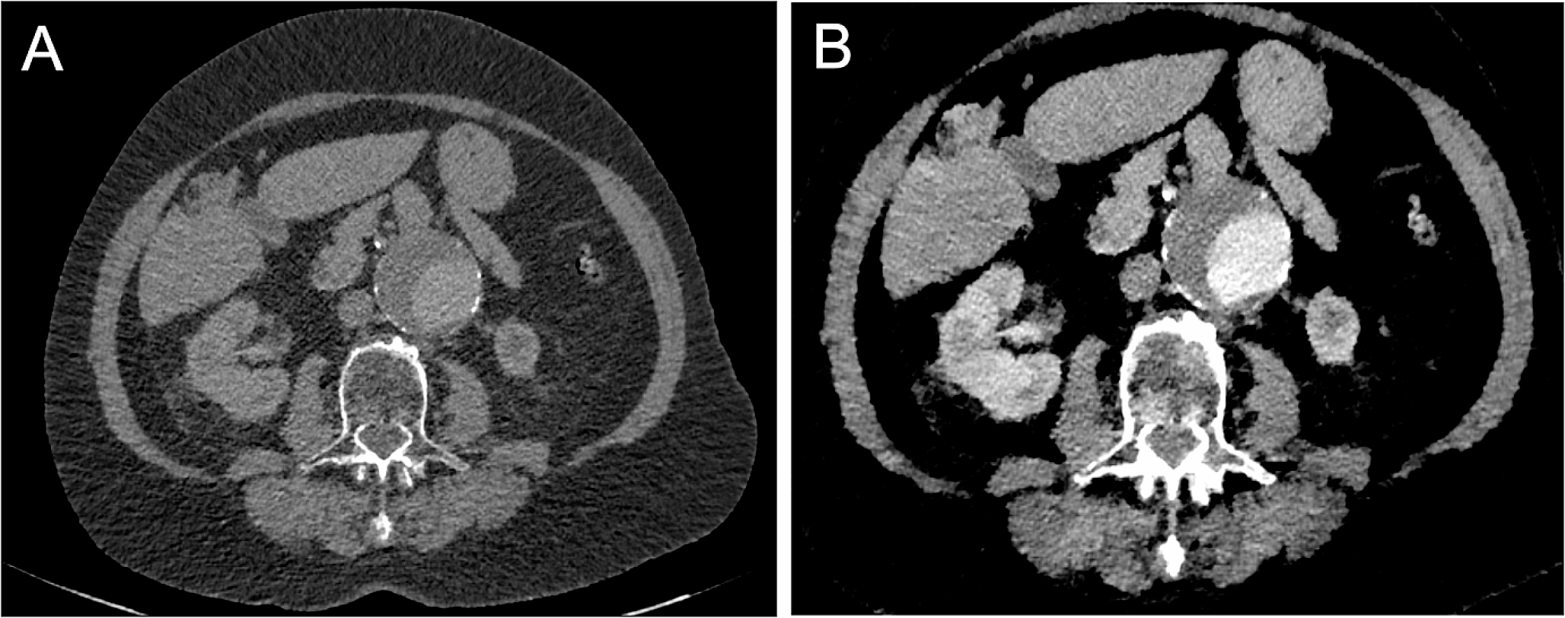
Conventional (A) and MonoE40 (B) axial reconstruction of a Gd-enhanced CT angiography of the abdominal aorta
